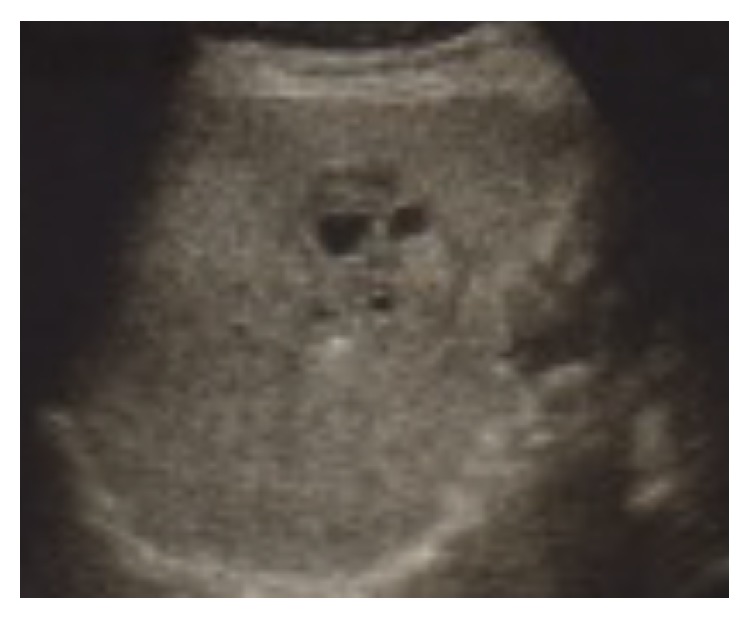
Ultrasonography (US) images. US showed an isoechoic nodule with small hypoechoic areas in segment 8 of the liver.
